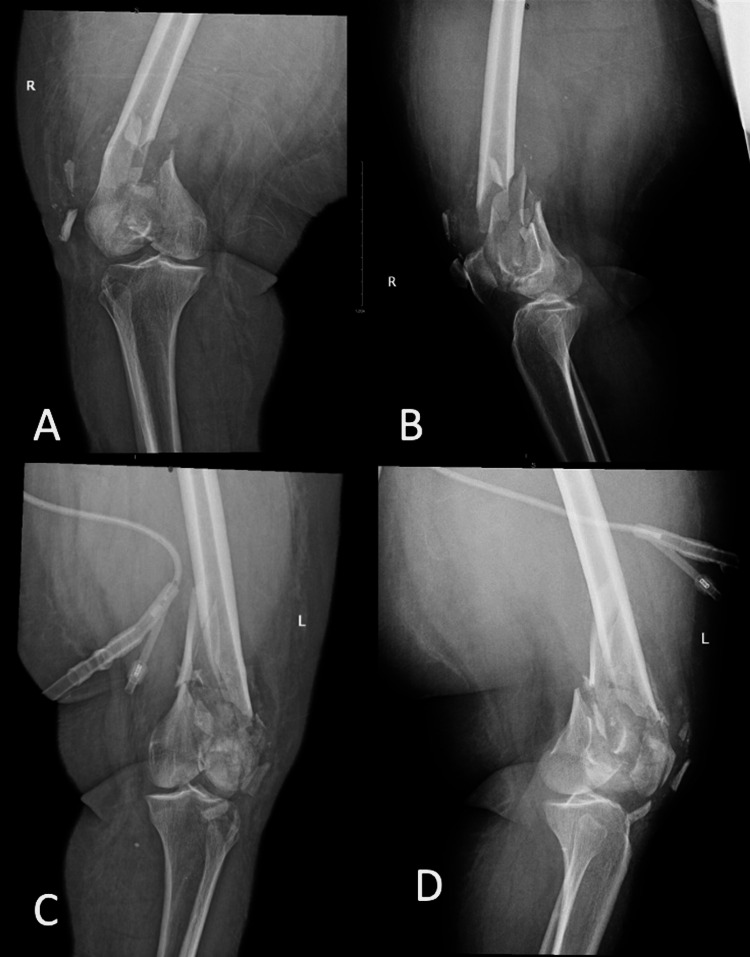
(A) Anteroposterior and (B) lateral radiographs of the right distal femur. (C) Anteroposterior and (D) lateral radiographs of the left distal femur.
